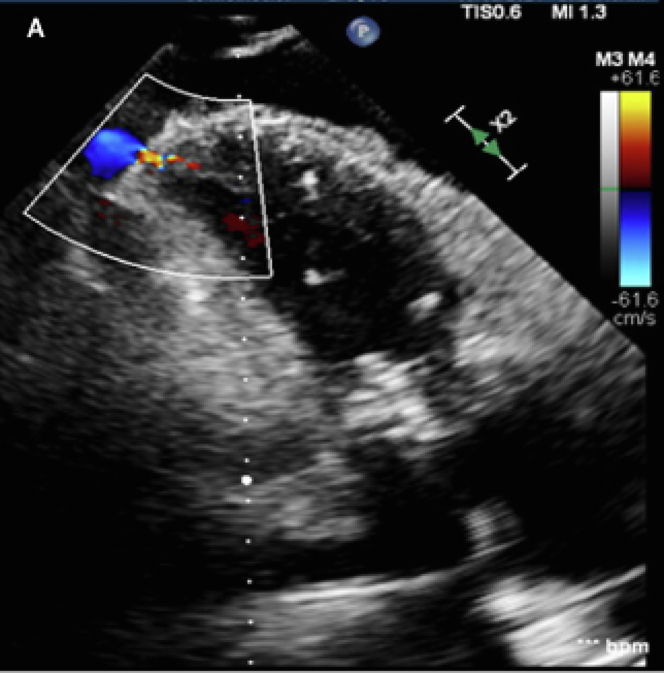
Left Ventricular Perforation Transthoracic echocardiogram with color Doppler showing perforation at the apex of the left ventricle.
